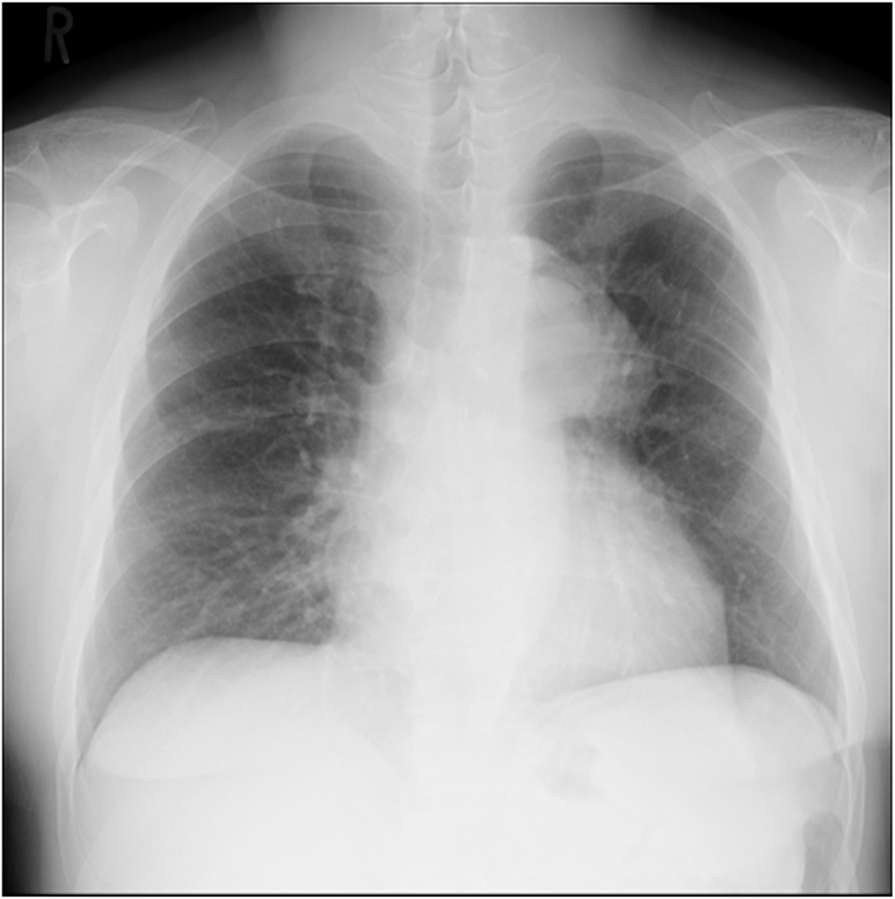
Preoperative chest radiography showing enlargement of the superior mediastinum
